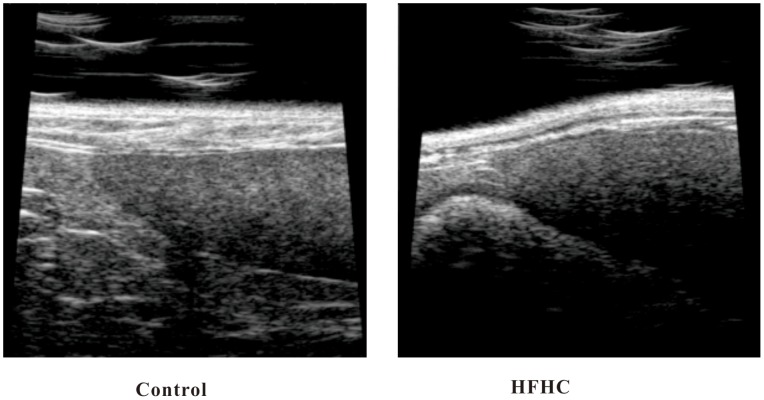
Ultrasonographic imaging of liver tissue from control (left) and HFHC hamsters (right) at week 42.
